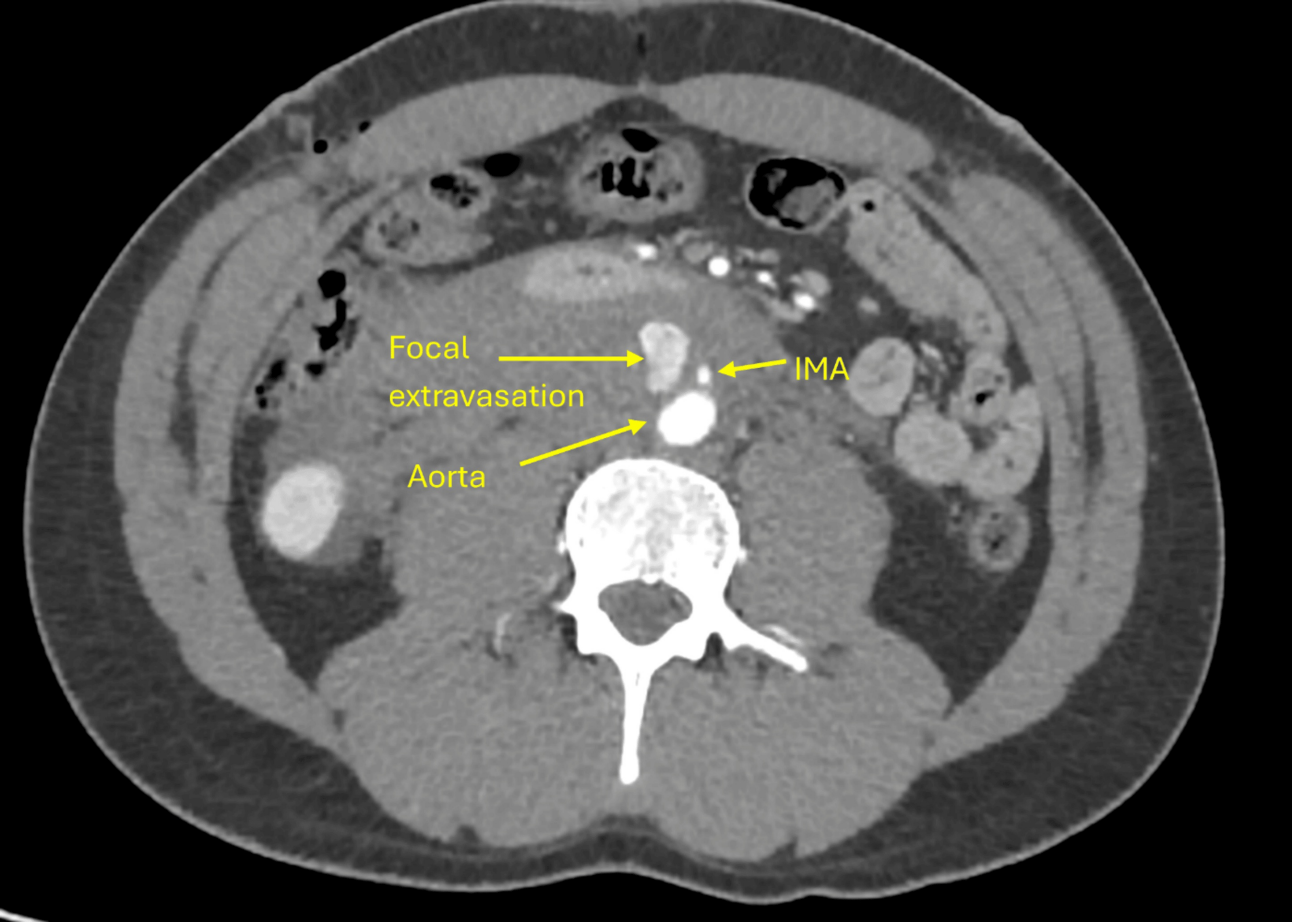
CT of the abdomen with contrast showing extravasation from the aorta with expanding hematoma at the level of the inferior mesenteric artery (IMA).
